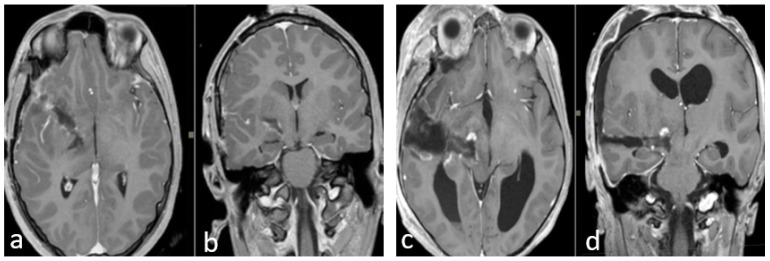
(a–d) Early postoperative brain MRI with gadolinium. (a,b) An early postoperative brain MRI, T1-weigthed with gadolinium in the axial (to the left) and coronal plane (to the right), for Case A in Figure 2. (c,d) An early post operative MRI, T1-weigthed with gadolinium, for Case B in Figure 1. In detail: In accordance with the nTMS data, we performed a transsylvian approach for Case A (a,b) obtaining a gross total resection (GTR). In Case B (b–d), we performed a trans-temporal approach, obtaining a subtotal resection (STR).
